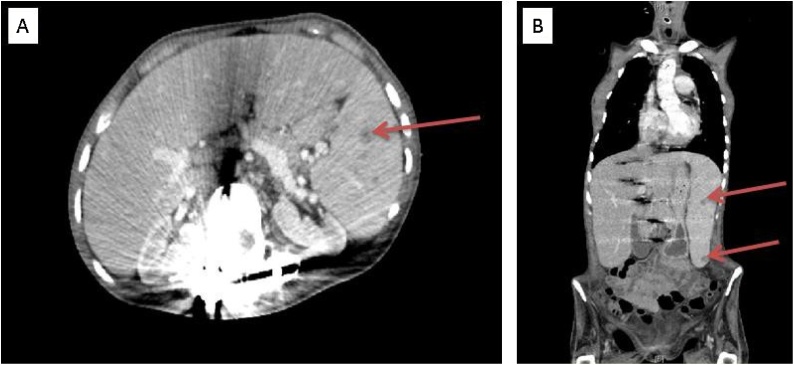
Computed tomography of the abdomen and pelvis with contrast and B shows the axial and coronal views respectively of computed tomography of chest, abdomen and pelvis demonstrating multiple ill-defined hypoattenuating lesions throughout an enlarged spleen.
